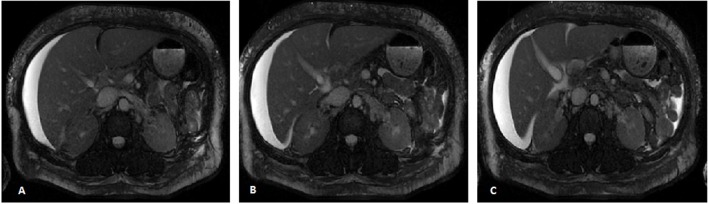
Three consecutive axial MR images, images A-C, of the abdomen demonstrating a normal appearing liver with moderate perihepatic ascites along with gallbladder wall thickening. Biliary dilatation is not demonstrated, with a normal pancreatic duct and a common bile duct measuring 3 mm.
